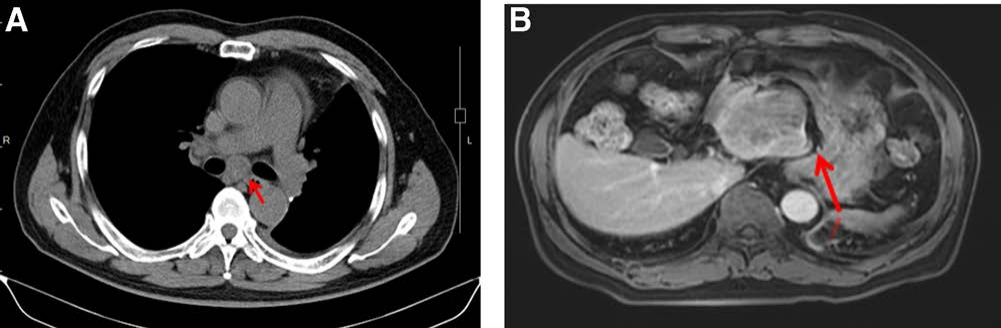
First radiological progression (May 2020). (A) Chest CT identified enlarged mediastinal lymph nodes (red arrow; 2.6 cm). (B) Abdominal MRI showed interval growth of the left-lobe mass to 7.3 cm × 6.0 cm (red arrow). CT = computed tomography, MRI = magnetic resonance imaging.
